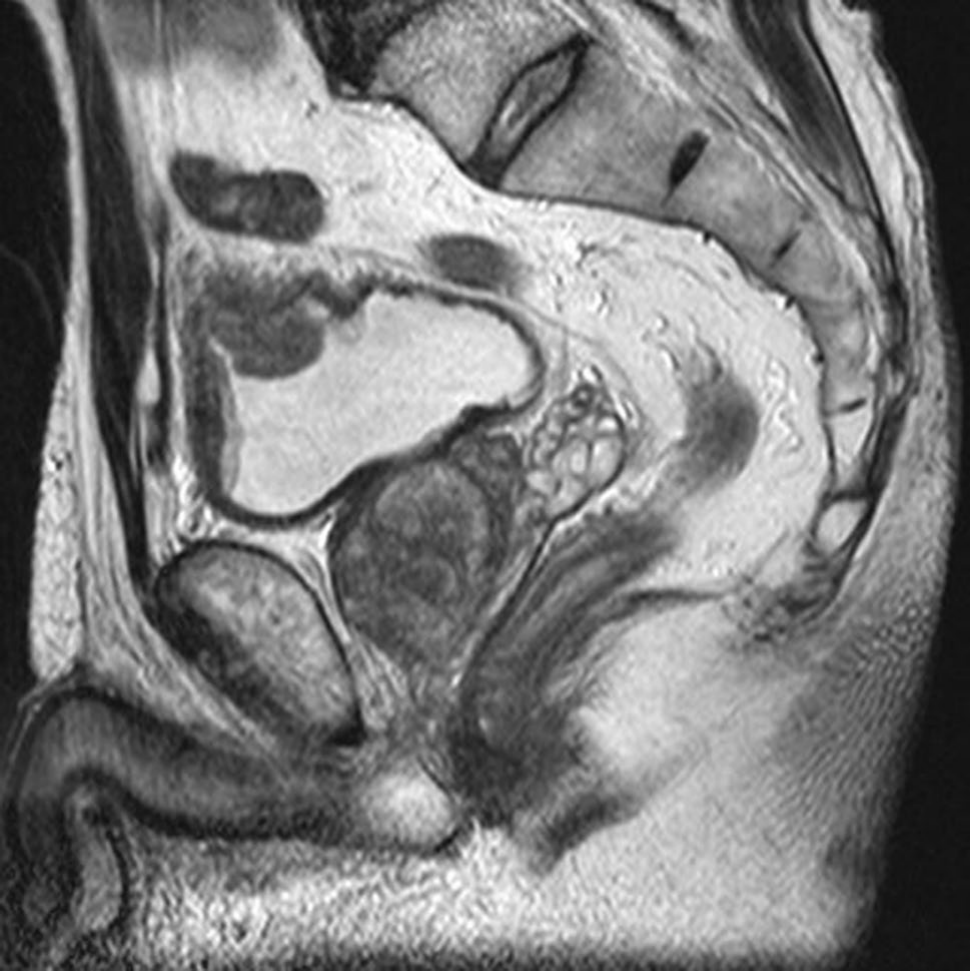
MRI after transurethral resection of bladder tumor. The bladder tumor was suspected to have invaded the muscle layer
